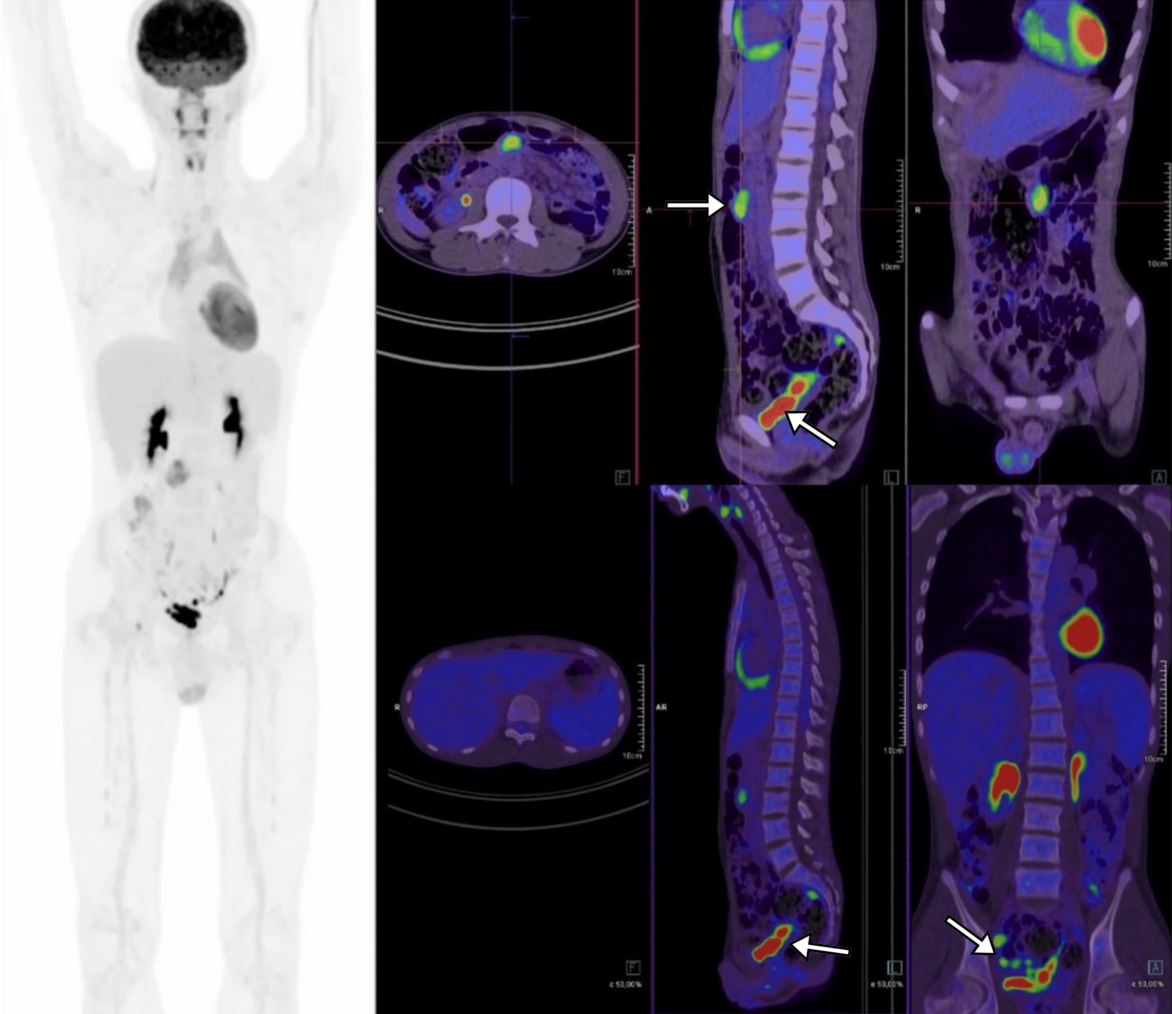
FDG PET/CT follow-up scan after the fourth cycle of chemotherapy showed residual lymphadenopathies (white arrows) in the mesentery, pelvis, and left and right para-aortic areas. No residual masses were found at the right frontal, temporal, intraorbital, and costal regions. Additionally, no involvement of the liver, bones, bone marrow, or other organs was found.
